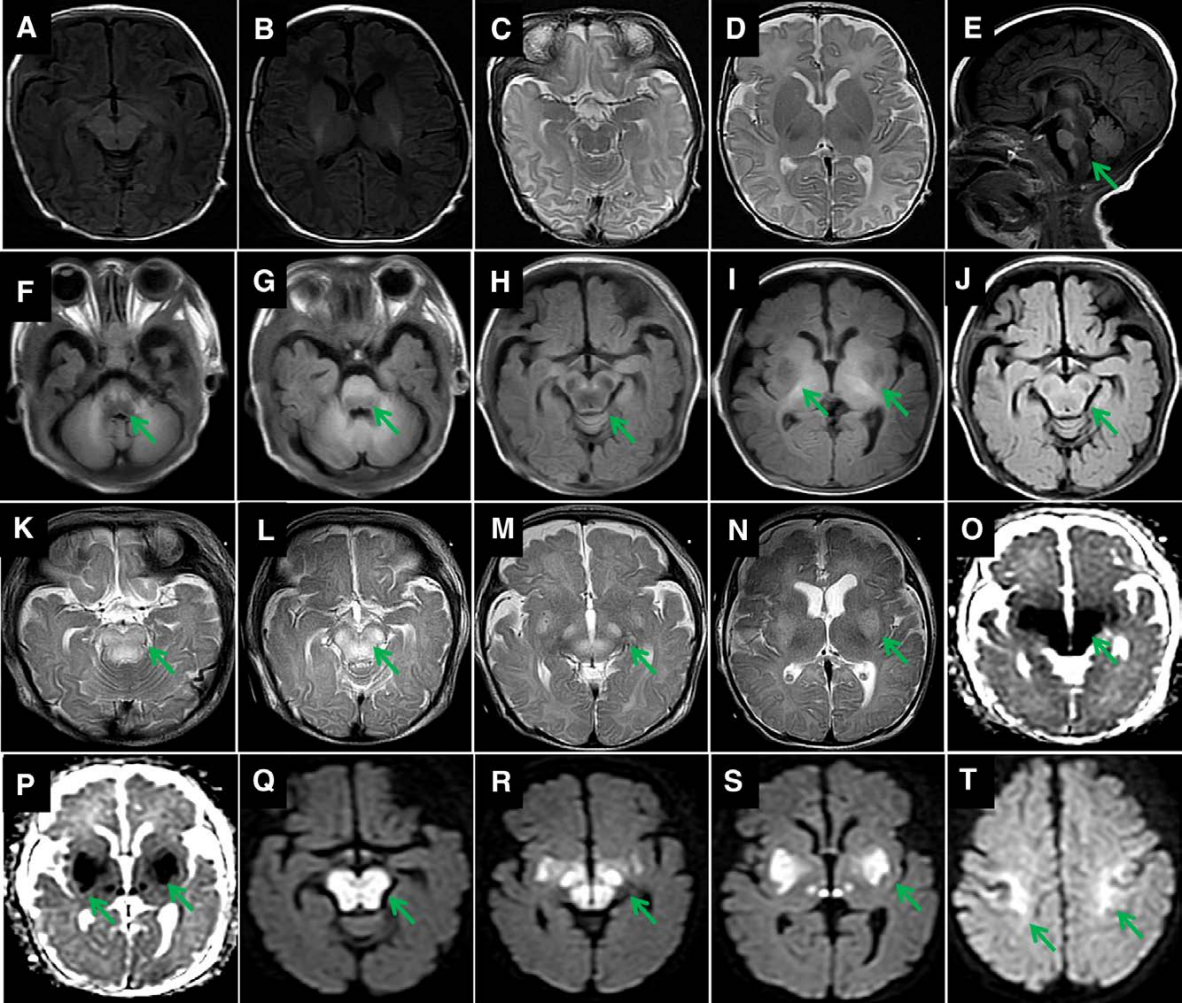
Evolution of lesions on MRI. Brain MRI (A, B, C, and D) on day 8 showing no obvious abnormalities. MRI on day 30 showing symmetrical lesions in the bilateral basal ganglia, cerebral peduncle, and brainstem hypointense on T1-WI (E, F, G, H, and I, arrows), fluid-attenuated inversion recovery imaging (J, arrow), apparent diffusion coefficient (O and P, arrows), hyperintensity on T2-WI (K, L, M and N, arrows), and diffusion limitation on diffusion WI (Q, R, S and T, arrows). MRI = magnetic resonance imaging, WI = weighted imaging.
